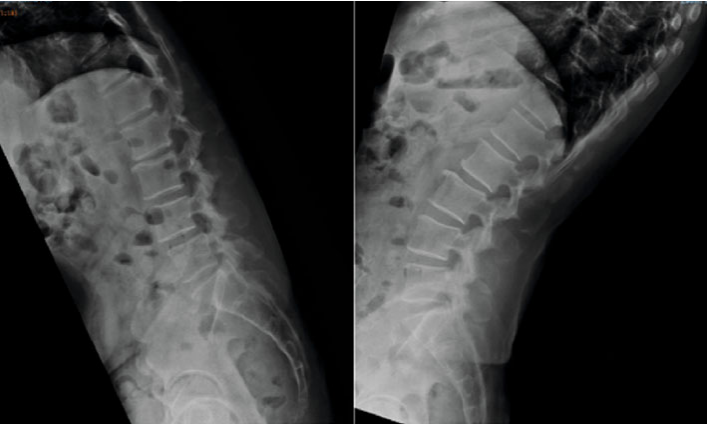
Preoperative X-ray films. No further instability of L4 over L5 is evident in upright lateral flexion and extension views.
